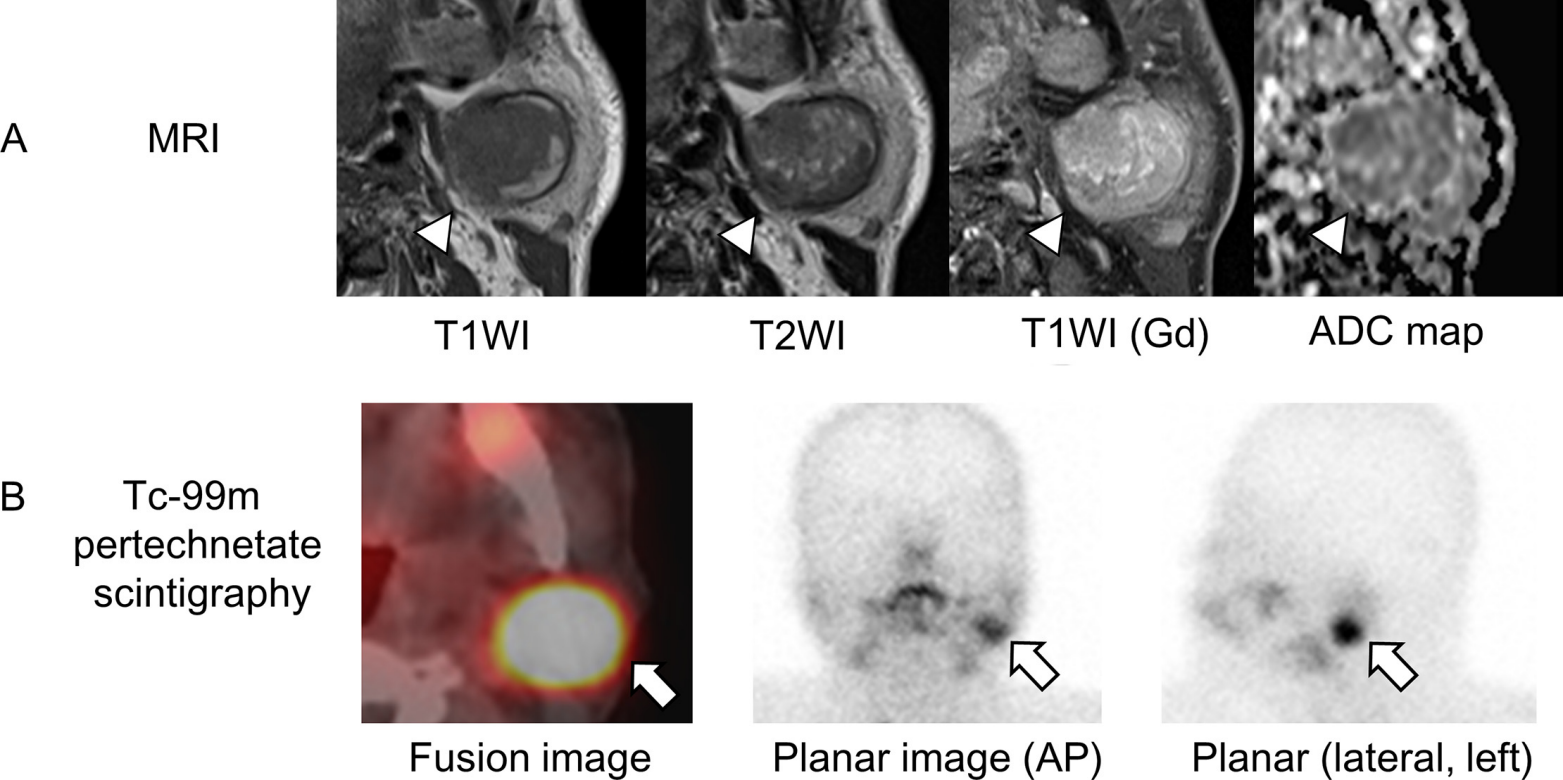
MRI and ^99m^Tc pertechnetate scintigraphy in a representative case of Warthin tumor. Warthin tumor on the left side of a 60-year-old man (case 14). (A) Axial MRI (white arrowheads) showed heterogenous (low to high) intensity on T1WI, heterogenous (low to intermediate) intensity on T2WI, strong gadolinium enhancement, and a low signal on the ADC map (ADC_mean_ 0.94 × 10^−3^ mm^2^/sec). (B) A fusion image (bottom left) and planar scans (bottom middle and right) in ^99m^Tc pertechnetate scintigraphy showed intense uptake (white arrows) in the tumor.
